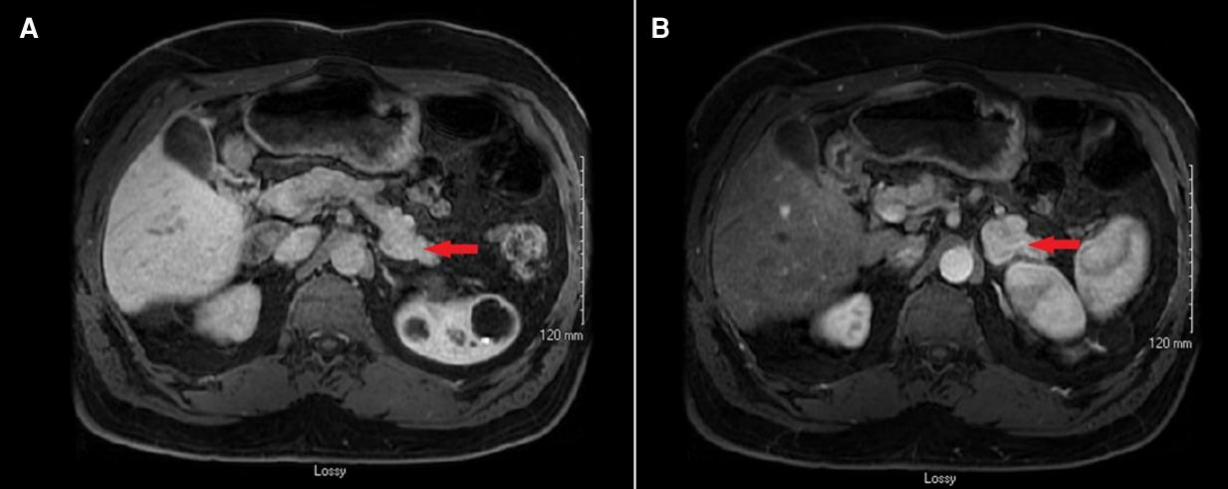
(A) Computed tomography image of the abdomen, demonstrating a large heterogeneous enhancing insulinoma arising from the tail of the pancreas. (B) Magnetic resonance imaging scan of the abdomen revealing of a mass in the tail of the pancreas, which represents neuroendocrine tumor/insulinoma.
